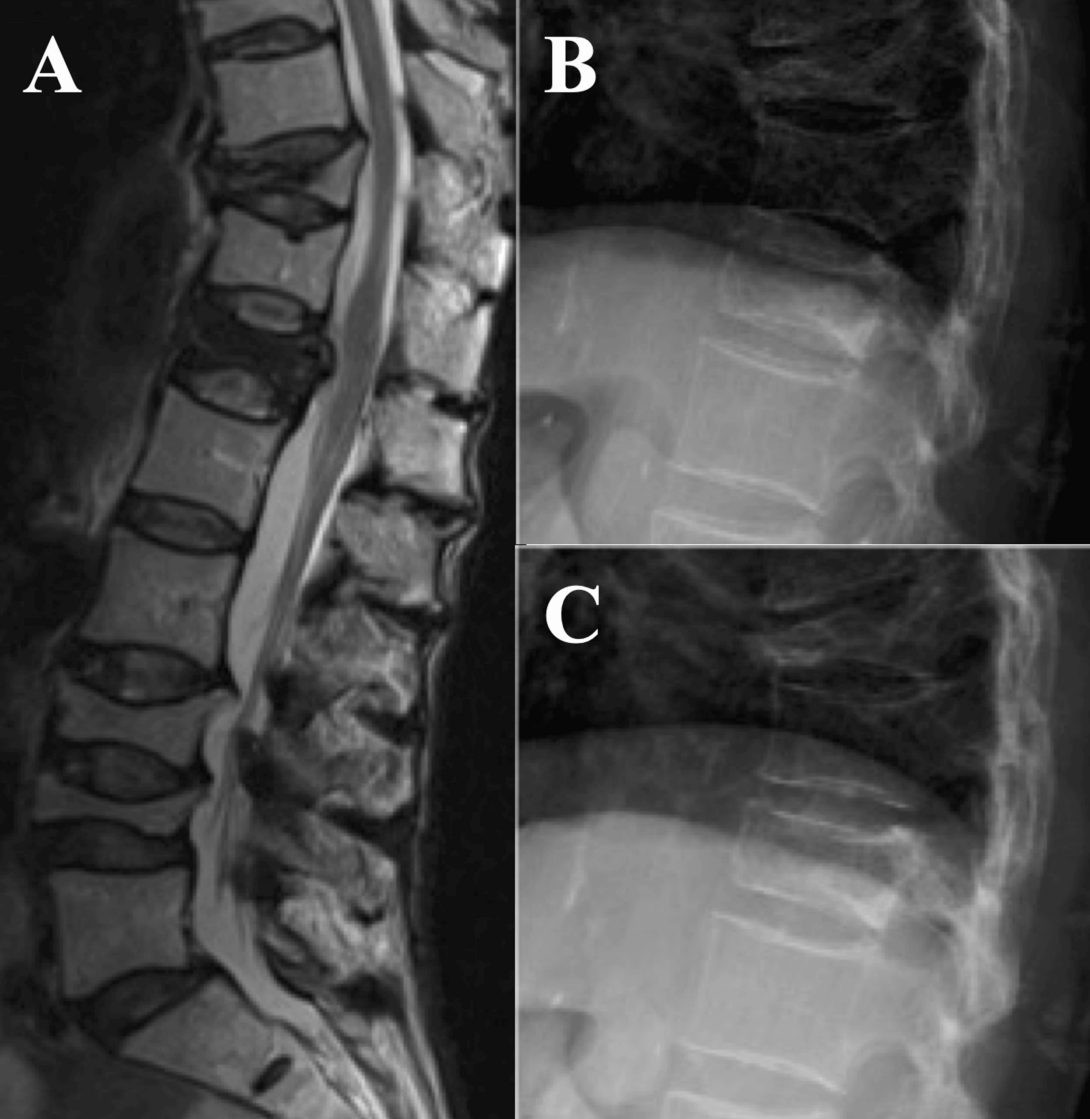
Imaging findings of the thoracic and lumbar vertebrae A: Sagittal T2-weighted MRI on admission showing T12 vertebral fracture and T10, L3, and L4 chronic OVF. B: Standing X-ray. C: Supine X-ray showing slight intravertebral instability. OVF, osteoporotic vertebral fractures
